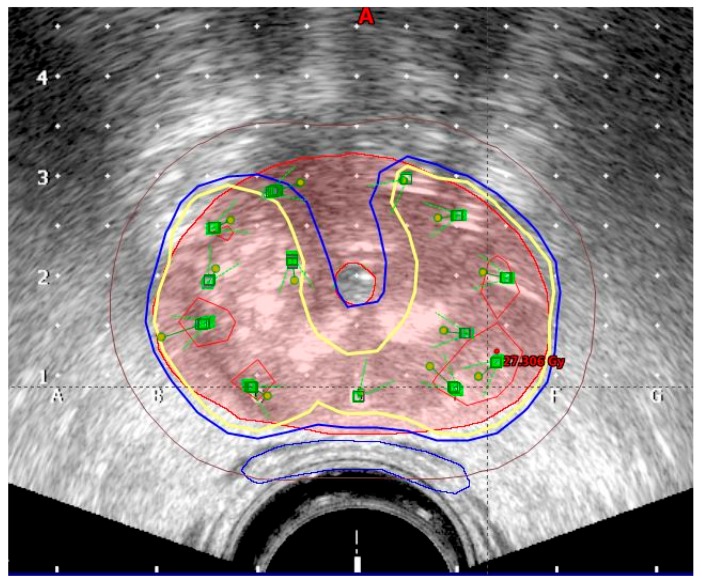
Isodoses in transrectal ultrasound image (red: 15 Gy; yellow: 9 Gy; blue: 8 Gy; brown: 5 Gy).
